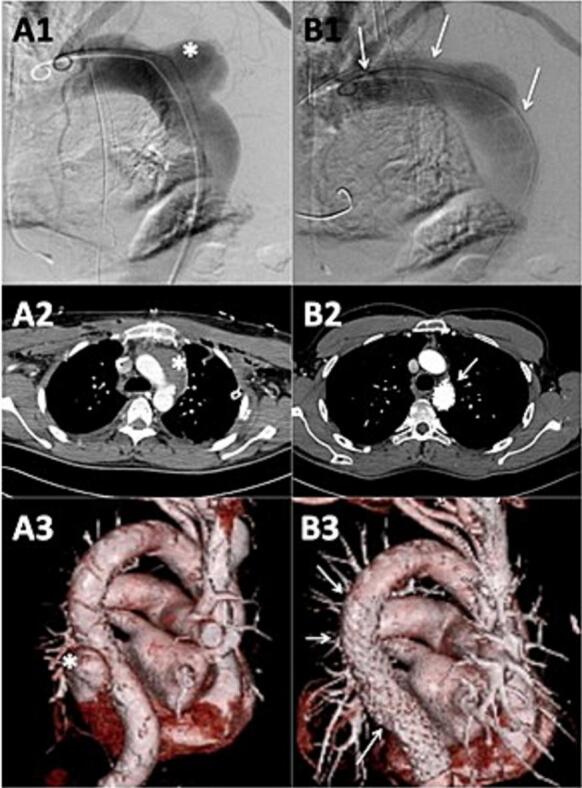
Patient with non-traumatic rupture before (A) and after (B) thoracic endovascular aortic repair. Shown are the pre- and postprocedural results in the angiogram (A1, B1), 2D Computed tomography (CT) −scan (A2, B2) and 3D CT reconstruction (A3, B3). The thoracic rupture spot (*) is treated by an endovascular approach through an aortic stent graft (arrow).
